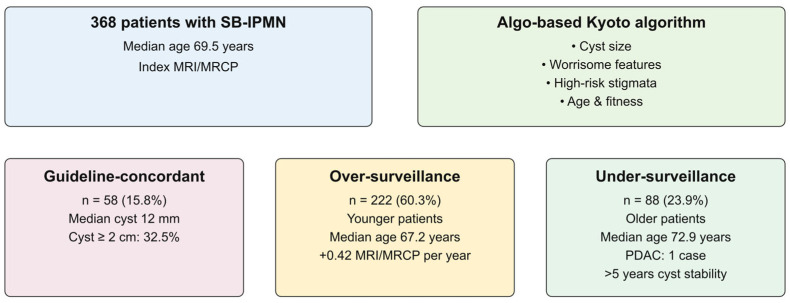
AIgo-based comparison of real-life SB-IPMN management versus Kyoto guidelines. Most SB-IPMN patients (60.3%) were managed more intensively than recommended, particularly younger individuals with small, stable cysts. PDAC developed on one patient over 1661 patient-years. Excess imaging studies translated to an extra 0.42 MRI/MRCP per patient-year.
